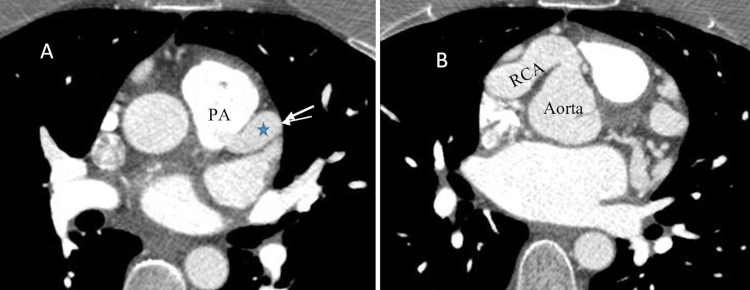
Axial projection of CCTA showing (A) LMCA (blue star) originating from the main pulmonary artery and (B) RCA in normal position and again much dilated. CCTA, coronary computed tomography angiogram; LMCA, left main coronary artery; RCA, right coronary artery
